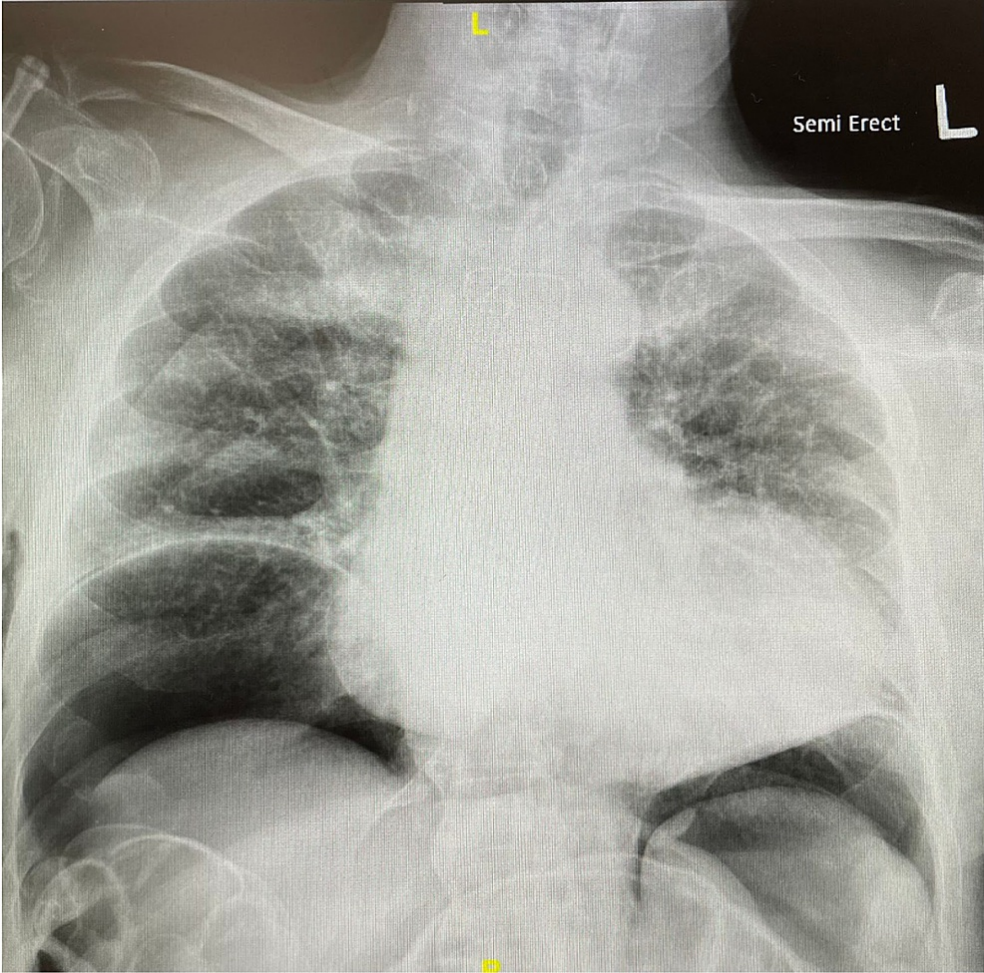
Admission CXR demonstrating pneumoperitoneum. CXR, chest X-ray
